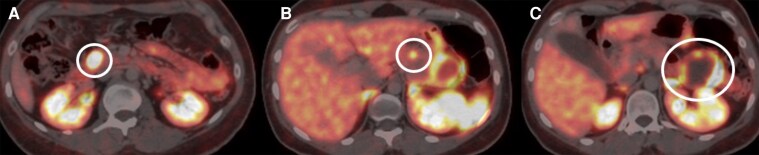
Axial views of ^68^Ga-DOTATOC PET/CT exhibiting neuroendocrine tumors on the head of the pancreas (A, white circle), body (B, white circle), and tail (C, white circle).
